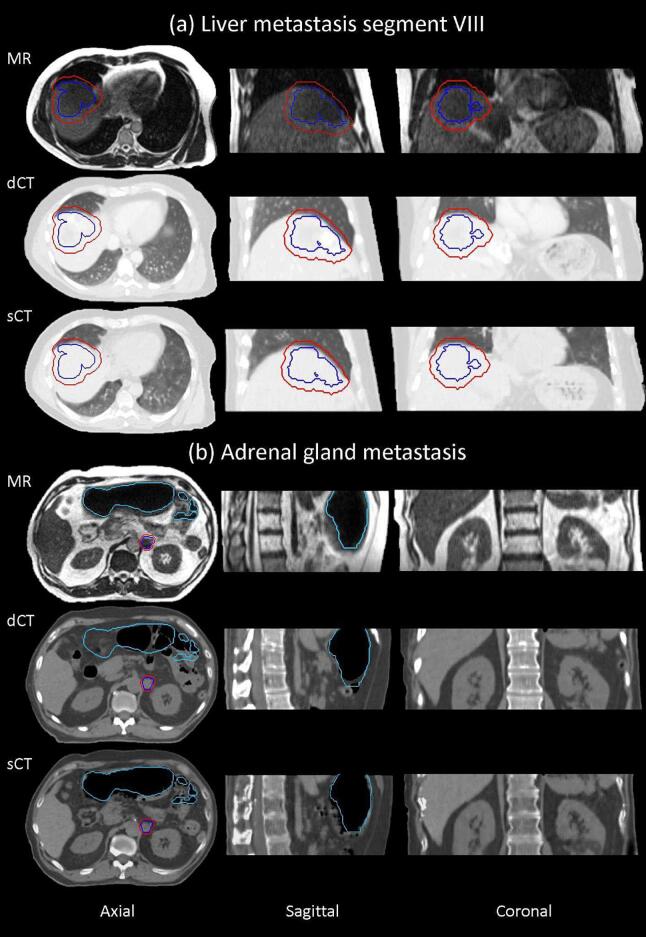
Example of sCT for a liver case (a) displayed in the lung CT window and an adrenal gland case (b) in soft tissue window. The contours of the PTV (red), GTV (blue) and air pockets in the MR image (cyan) are also displayed. The axial slices are located at the centre of the PTV (a, b) and the coronal and sagittal either at the centre of the PTV to show the lung tissue (a) or the vertebral bodies to show the bones (b). (For interpretation of the references to colour in this figure legend, the reader is referred to the web version of this article.)
